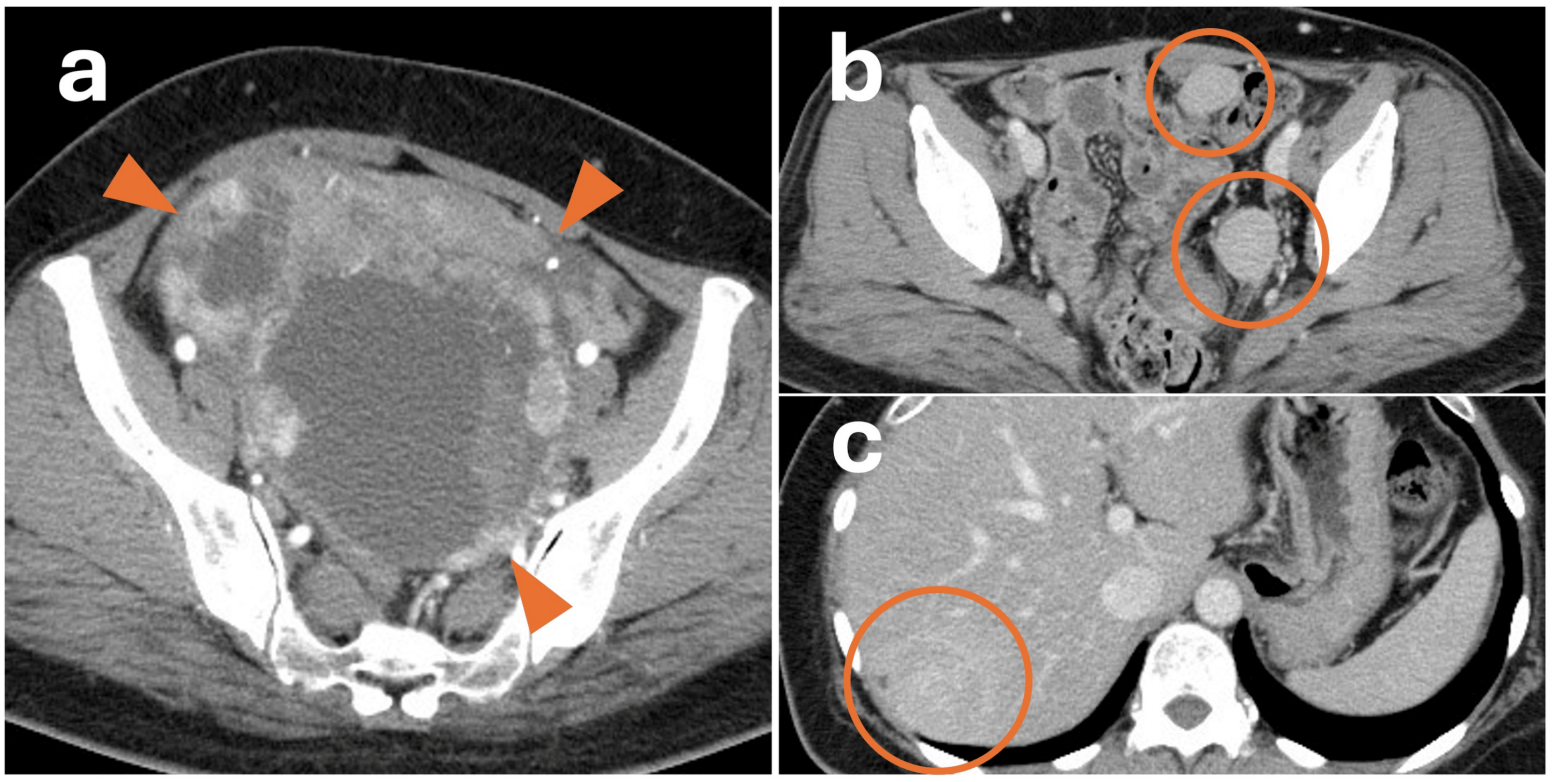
CT images of the tumor (a) Ovarian tumor occupying the pelvic cavity; (b) After surgery, enlargement of pelvic and (c) hepatic metastases was observed in association with rising thyroglobulin (Tg) levels (see also event #4 in Figure 1).
